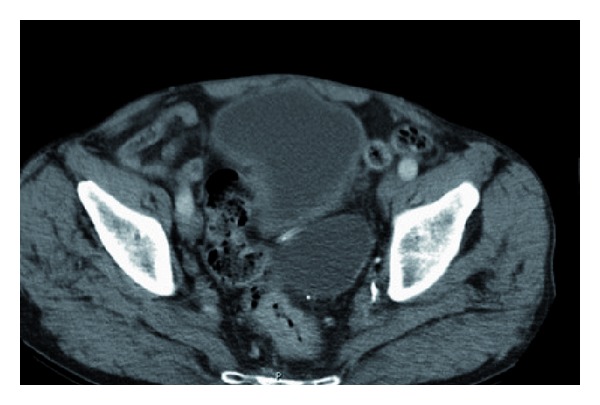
CT scan of a patient with obstructing carcinoma of the rectum.
